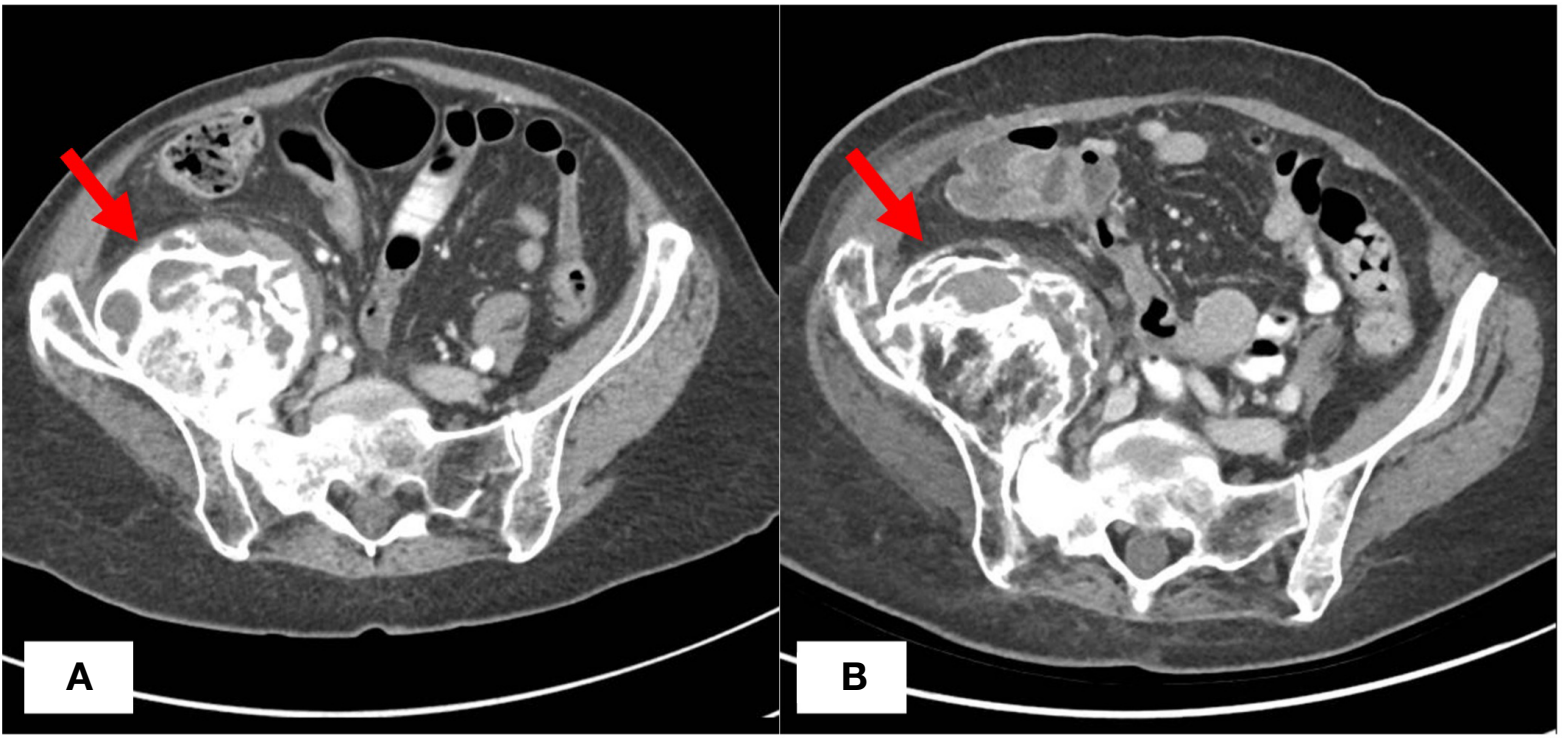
CT Imaging Before and After Treatment with Endocrine Therapy. CT abdomen/pelvis with IV contrast demonstrating largely unchanged right iliac mass before (A) and 57 months after (B) initiation of megestrol acetate and tamoxifen endocrine therapy.
